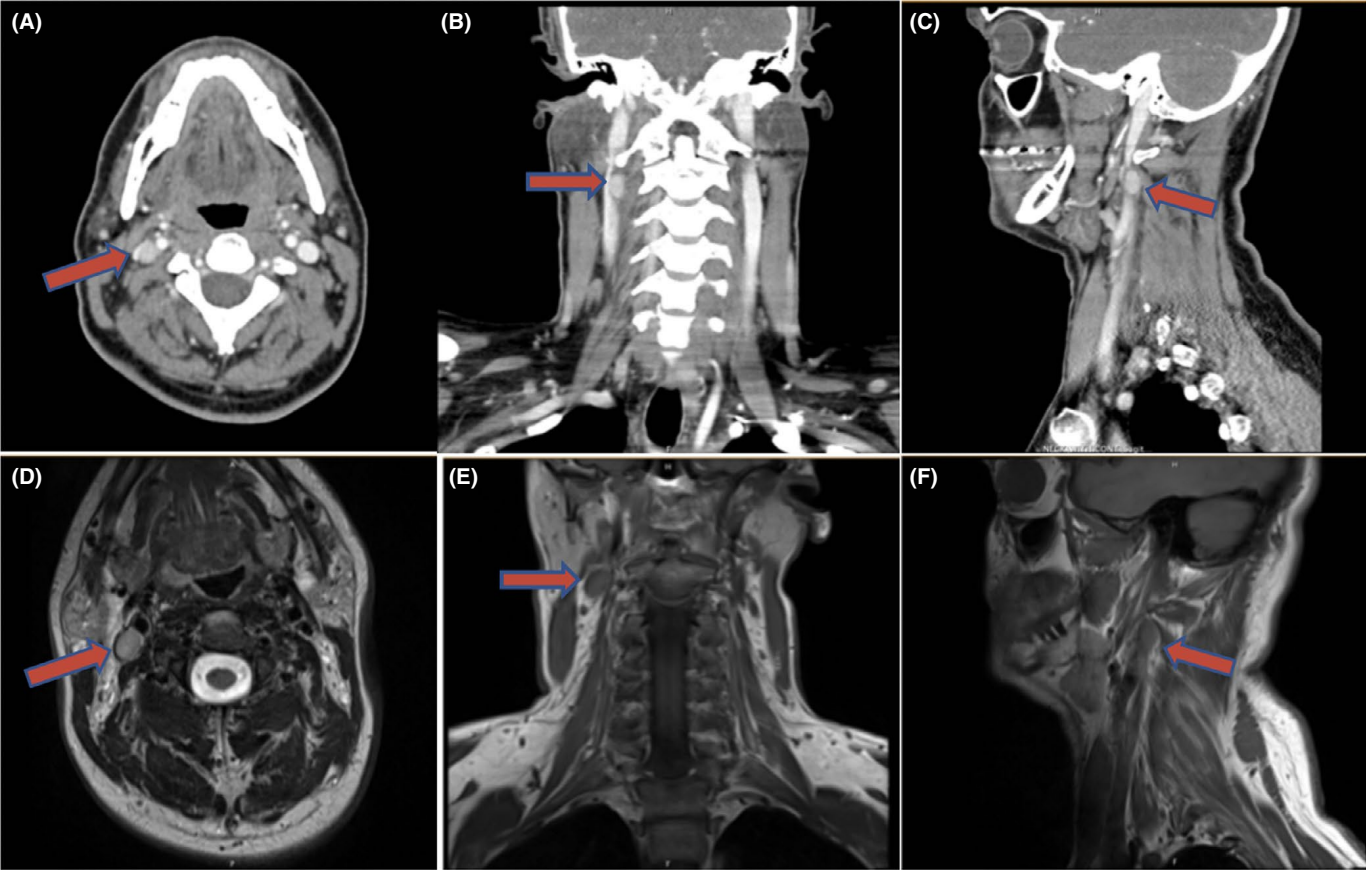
Corresponding CT and MRI images of intravagal parathyroid adenoma in Patient 2. A/D, Transverse cut demonstrating an ovoid mass at the level of the carotid bifurcation. B/E, Coronal view showing the relationship of the mass to the skull base. C/F, Sagittal plane demonstrating an ovoid mass within the carotid sheath
